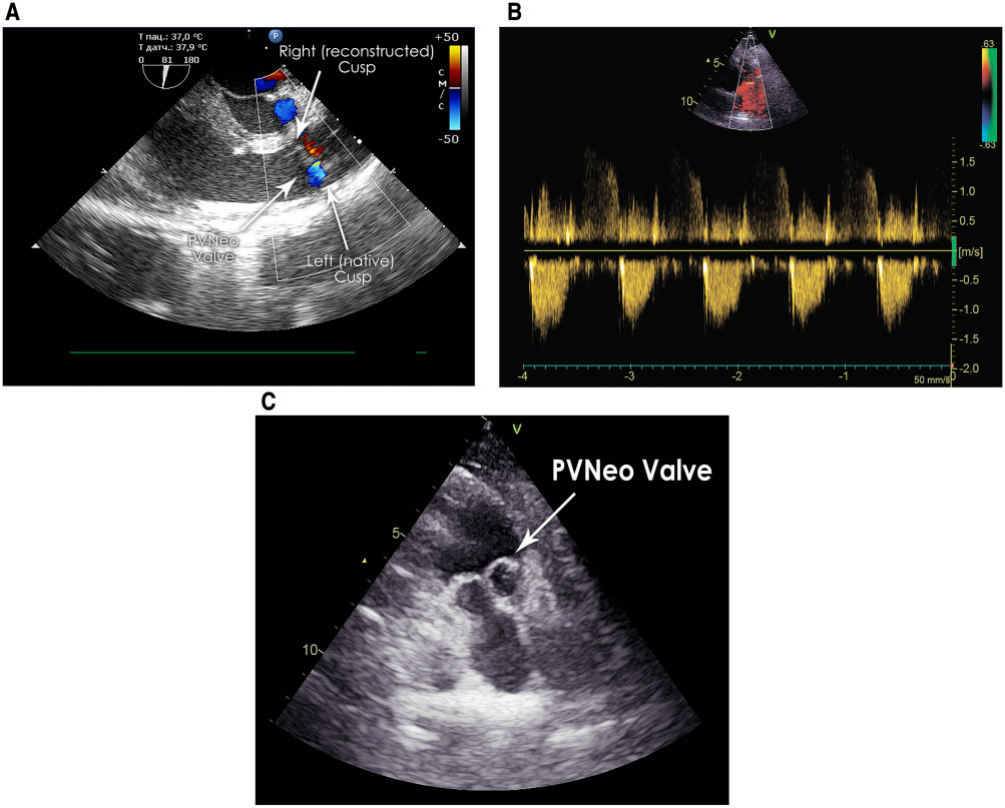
(A) Intraoperative transoesophageal echocardiography demonstrating good geometry and function of the reconstructed pulmonary valve in patient 1. (B) Colour Doppler image showing good haemodynamic performance of the same pulmonary valve neocuspidization after 2 years. (C) Transthoracic echocardiography (modified short-axis view) demonstrating good geometry and function of the pulmonary valve neocuspidization in patient 2 before discharge. PVNeo: pulmonary valve neocuspidization.
